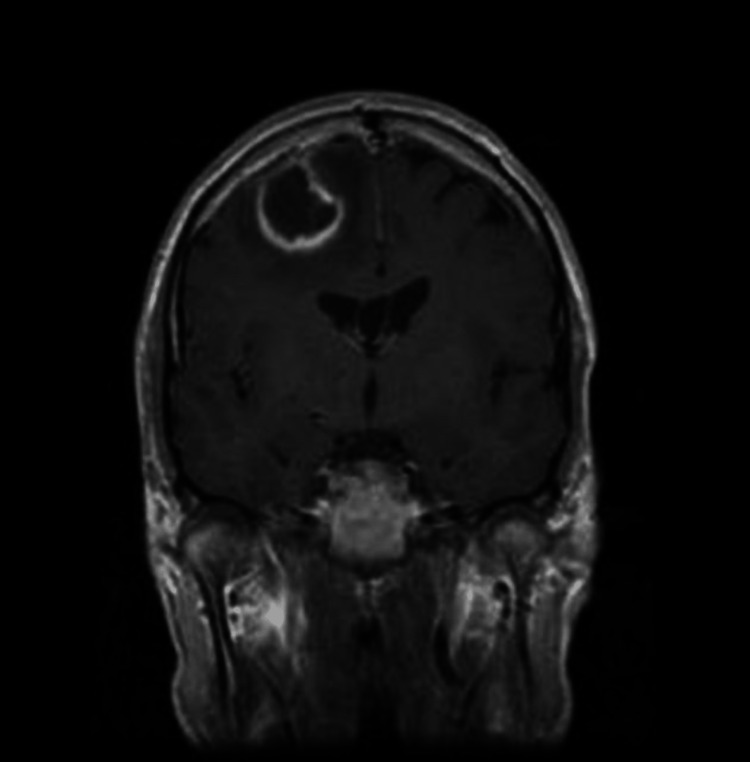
Magnetic resonance imaging (MRI) of the brain, a T1-weighted scan It reveals a 5.2 x 2.7 x 4.7 cm brain abscess in the temporoparietal region.
